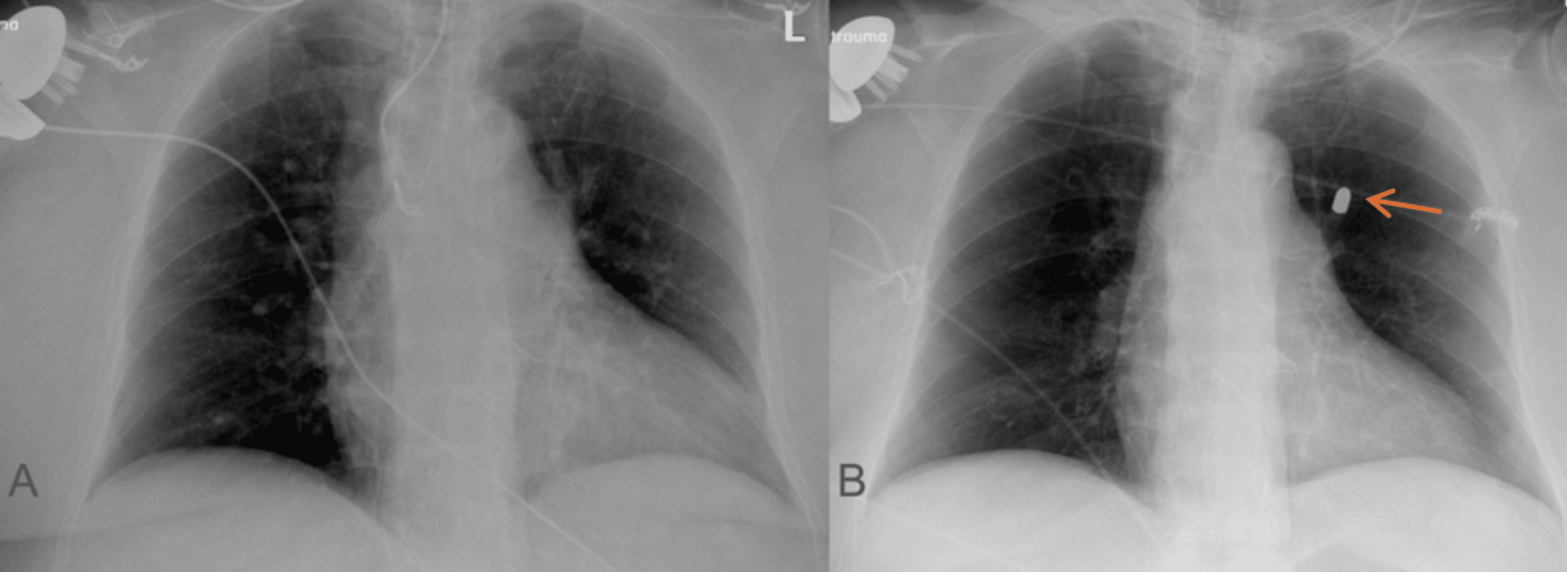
(A) A prior chest X-ray from one month before presentation depicting no evidence of a retained bullet. (B) A chest X-ray on initial presentation with evidence of a retained bullet in the left chest (orange arrow).
